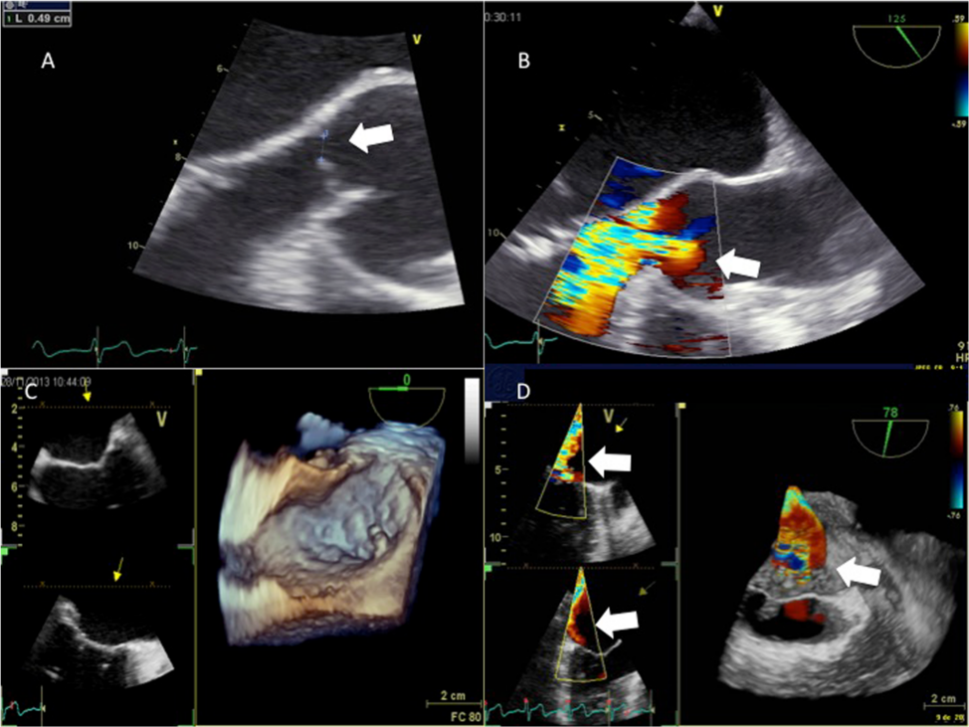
Transoesophageal echocardiographic views of the aortic and mitral valve. a Visualization of the perforation of aortic cusp (*arrow*). b Visualization of the large regurgitant jet through perforated aortic cusp (*arrow*). c Three-dimensional transoesophageal echocardiography of mitral valve. d Three-dimensional transoesophageal echocardiography: visualization of regurgitant color Doppler jet through mitral valve (*arrows*)
